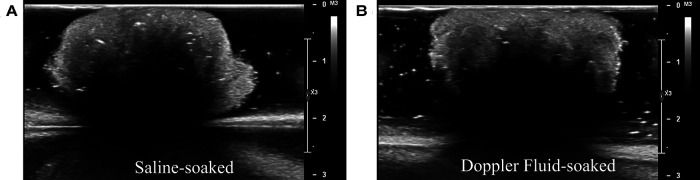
Acoustic comparison of cotton balls. (A) Experiments in this study were performed with saline-soaked cotton balls. (B) To ensure that cotton would not be visualized differently on an ultrasound machine when absorbing blood rather than saline, the saline-soaked cotton balls were compared to Doppler fluid-soaked cotton balls. Doppler fluid mimics the acoustic properties of blood. (A, B) are visually similar.
